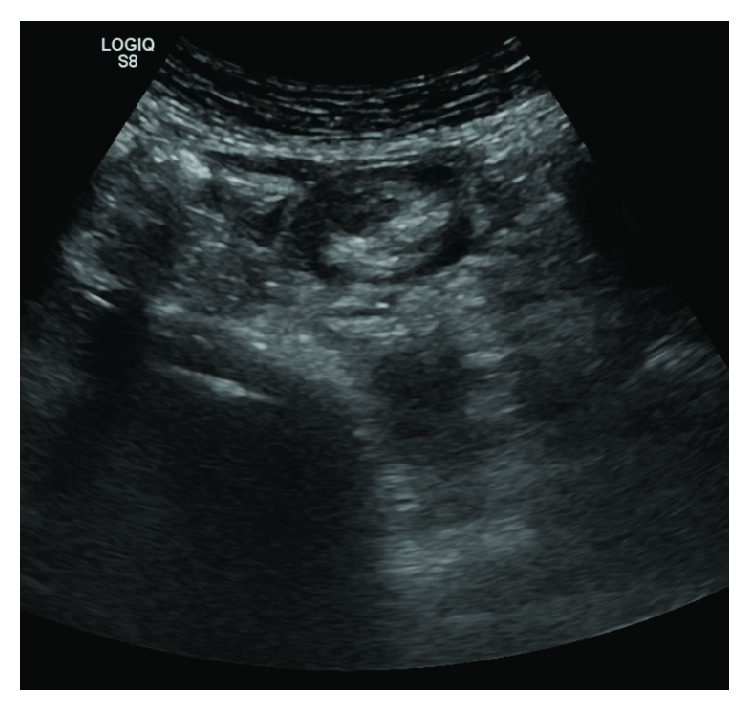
Abdominal ultrasound (possible intussusception).
